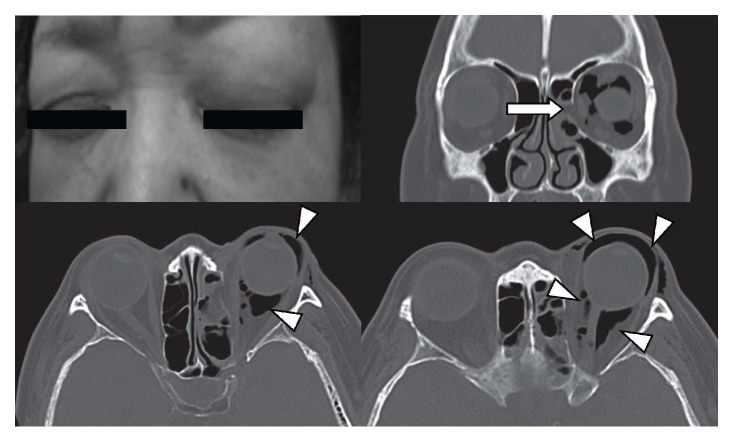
Nontender left periorbital swelling is noted (upper left panel). Computed tomography showed blowout fracture of the left orbital floor with orbital fat herniation (white arrow). Periorbital and orbital emphysema is noted (white arrowhead).
